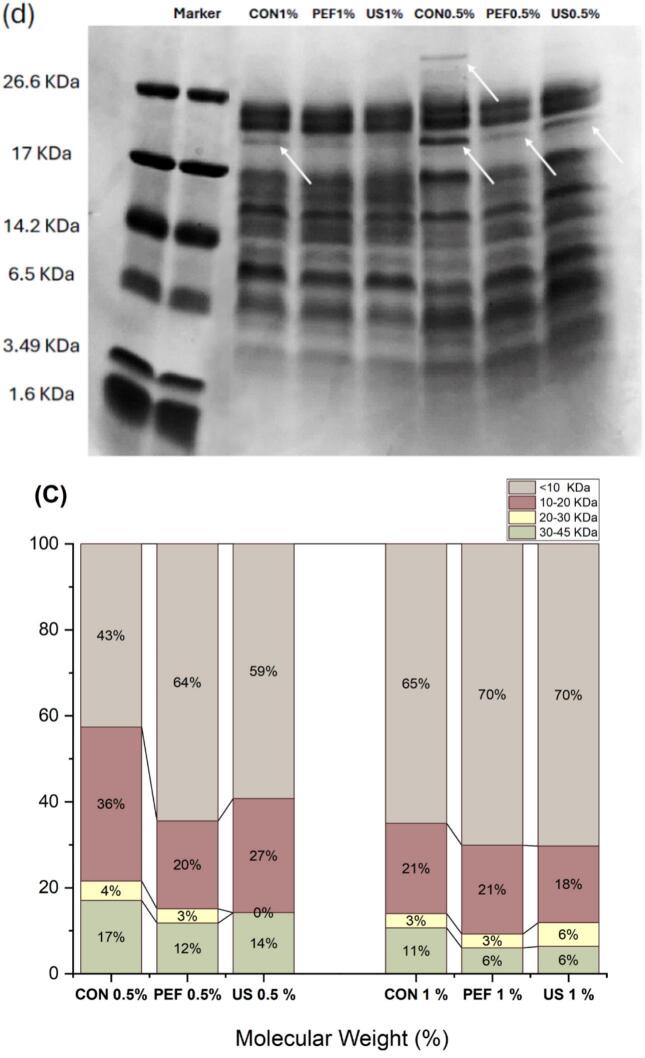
Size exclusion chromatograms showing the effect of ultrasonic (US) and pulse electric fields (PEF) pretreatment during enzymatic hydrolysis (a) with 0.5 % v/w and (b) 1 % v/w enzyme on molecular weight of the SFCB hydrolysates, (c) their relative percentage of total peak area and (d) their SDS-PAGE. CON 0.5 %: Conventional hydrolysis with 0.5 % enzyme, US0.5 %: ultrasound pretreatment followed with hydrolysis with 0.5 % enzyme, PEF0.5 %: pulsed electric field pretreatment followed with hydrolysis with 0.5 % enzyme. CON 1 %: Conventional hydrolysis with 1 % enzyme, US 1 %: ultrasound pretreatment followed with hydrolysis with 1 % enzyme, PEF 1 %: pulsed electric field pretreatment followed with hydrolysis with 1 % enzyme. In the SDS-PAGE gel, 10 μl of samples were loaded to each lane.
